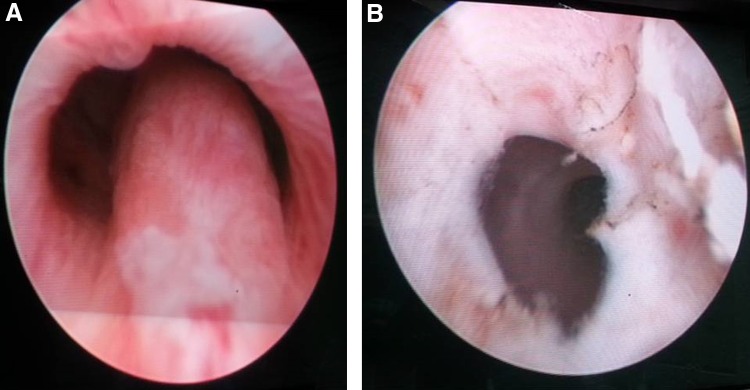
(A) Coronal section of a contrast-enhanced abdominal computed tomography showing single right kidney, left kidney agenesis, and right-sided seminal vesicular cystic dilatation. (B) Axial section single right kidney with evidence of compensated hypertrophy.
